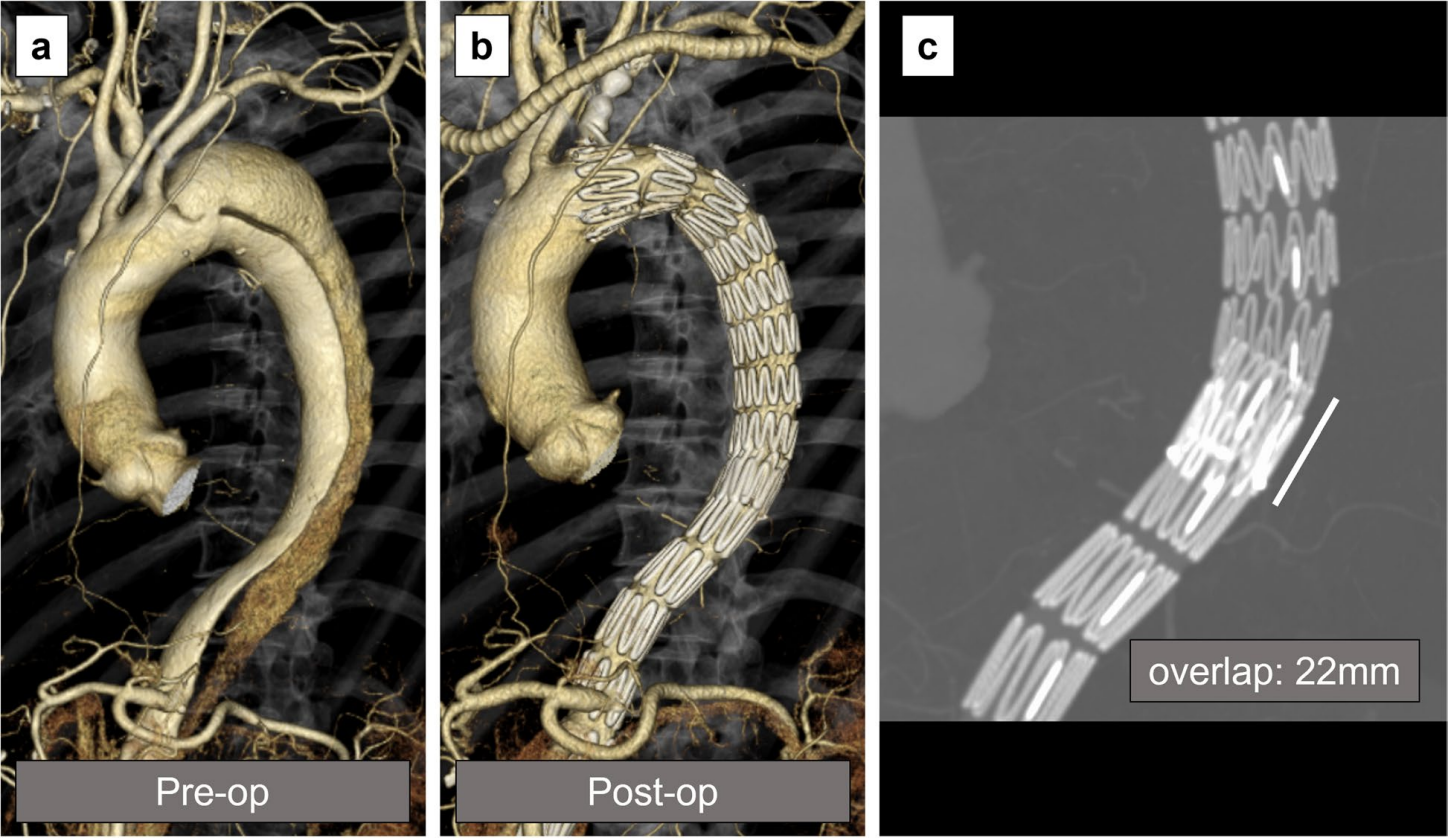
Pre- and postoperative computed tomography (CT) images of the first thoracic endovascular aortic repair (TEVAR). a Distal aortic arch aneurysm with type B aortic dissection; 3 years had passed since the onset. b Postoperative CT scan of the first TEVAR. c The length of the overlap with the stent graft and bare-metal stent at the first TEVAR
